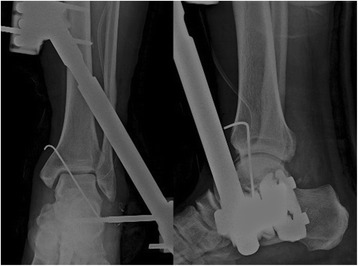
After immediate reimplantation of the extruded talus, its position around the ankle joint was maintained using a single K-wire fixation and spanning external fixator. Postoperative radiographs show the anatomical reduction of the ankle joint, including the subtalar joint and tibiotalar joint
